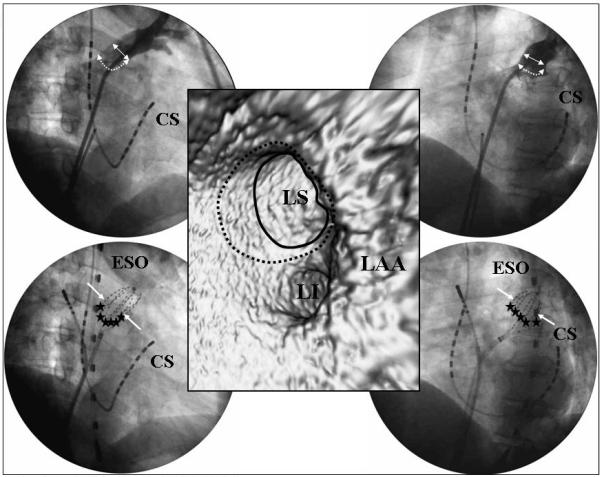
The relationship between the PV antrum and MBC. The upper panels show a selective angiogram of the LSPV and lower panels the position of the MBC. The left panels show the RAO view and right panels the LAO view. The middle panel shows the virtual endoscopic image of the left PVs. The solid arrows and circle indicate the PV ostium, dotted arrows and circle the PV antrum, and stars the PV antrum ablation sites. CS=coronary sinus, ESO=the transesophageal lead, LAA=left atrial appendage, LI=left inferior PV, LS=left superior PV. Reprinted from Yamada T et al. Electrophysiological pulmonary vein antrum isolation with a multielectrode basket catheter is feasible and effective for curing paroxysmal atrial fibrillation: efficacy of minimally extensive pulmonary vein isolation. Heart Rhythm 2006;3:377-384. Copyright (2006), with permission from The Heart Rhythm Society.
